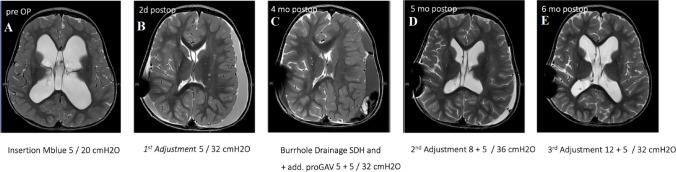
MRI T2-weighted images of a 2-year-old girl taken at different time points, demonstrating ventricular changes and subdural hematoma development over time due to shunt OD and a complex clinical course
